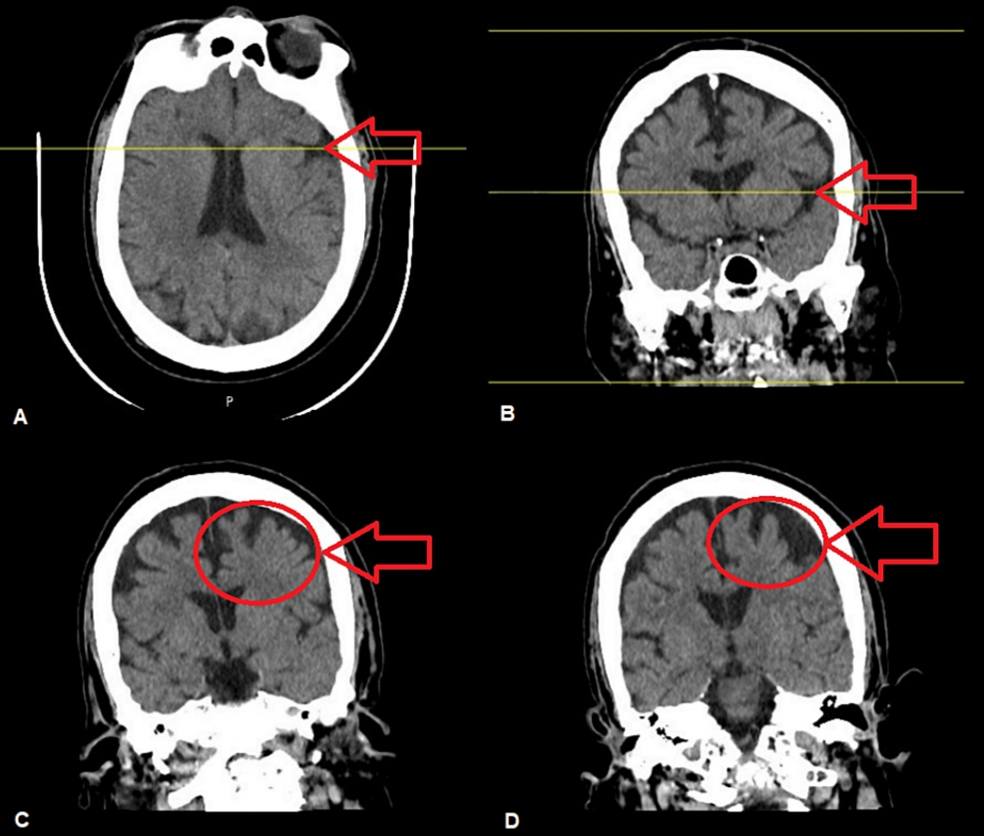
Cranial non-contrasted computed tomography scan. (A, B) Axial-coronal views showing left temporal atrophy (axial-coronal alignment shown by the yellow line). (C, D) Coronal view showing predominant left frontal lobe atrophy.
